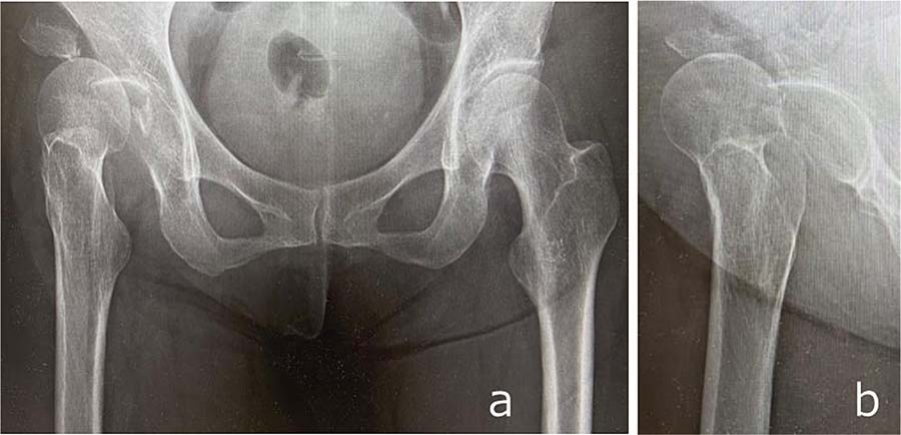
Anteroposterior (a) and lateral (b) radiographs of the right hip obtained at initial presentation, demonstrating anterior hip dislocation associated with acetabular and anterior inferior iliac spine fractures.
